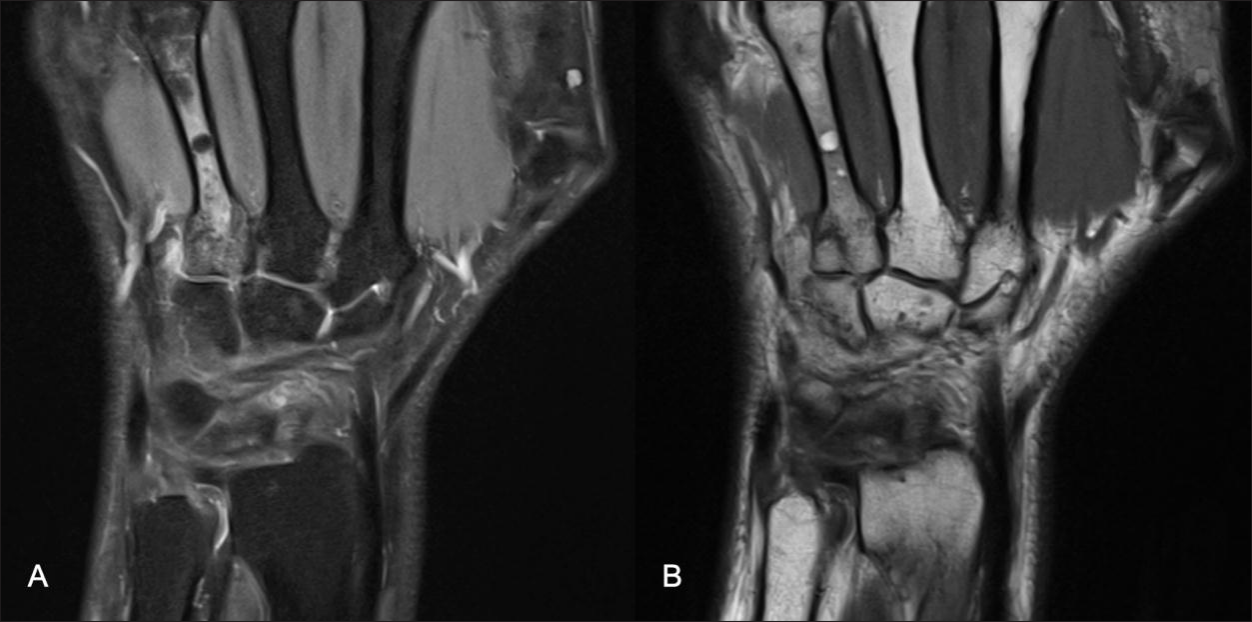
A dual-energy computed tomograpy (DECT) scan was performed to confirm the diagnosis. No fracture line was seen. Water reconstructions of the patient’s right hand showed massive bone marrow edema in metacarpal IV (Figure 2A). Fat reconstructions confirmed small fat globules at the diaphyseal level and an associated relative absence of fatty yellow marrow elsewhere in the bone (Figure 2B).
